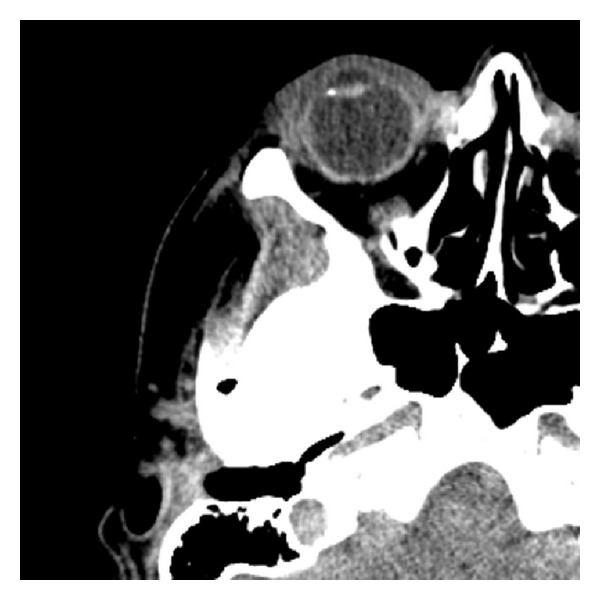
A noncontrast CT scan demonstrating foreign body resting on the zonules area.
